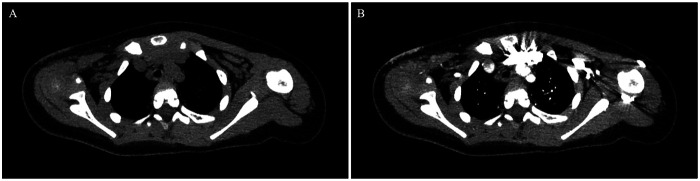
A girl with tufted angioma, aged 1 year and 2 months. (A) Plain CT showing cutaneous flocculent soft-tissue shadows and punctate soft-tissue intensity within subcutaneous adipose tissue. (B) Contrast-enhanced CT showing heterogeneous enhancement. CT, computed tomography.
